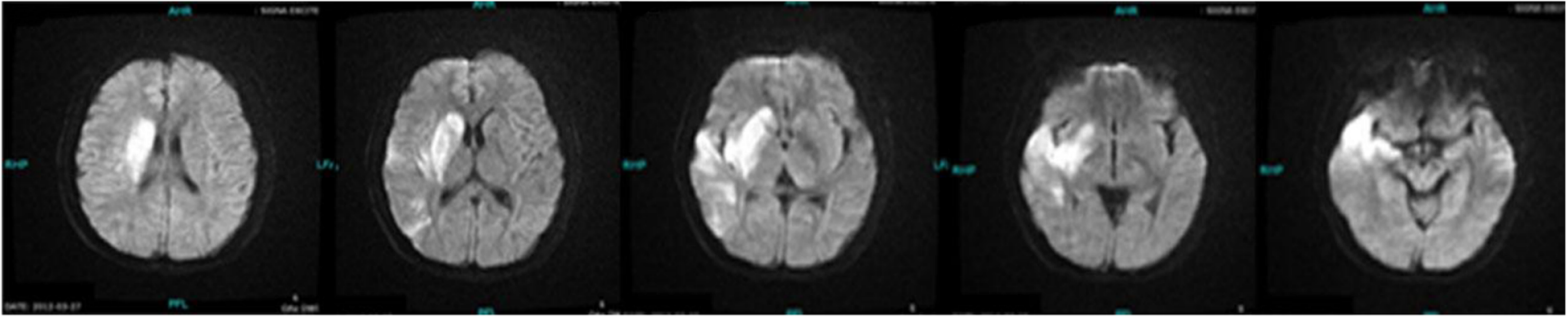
Brain magnetic resonance imaging (MRI) scan showing massive acute ischemic infarct.
